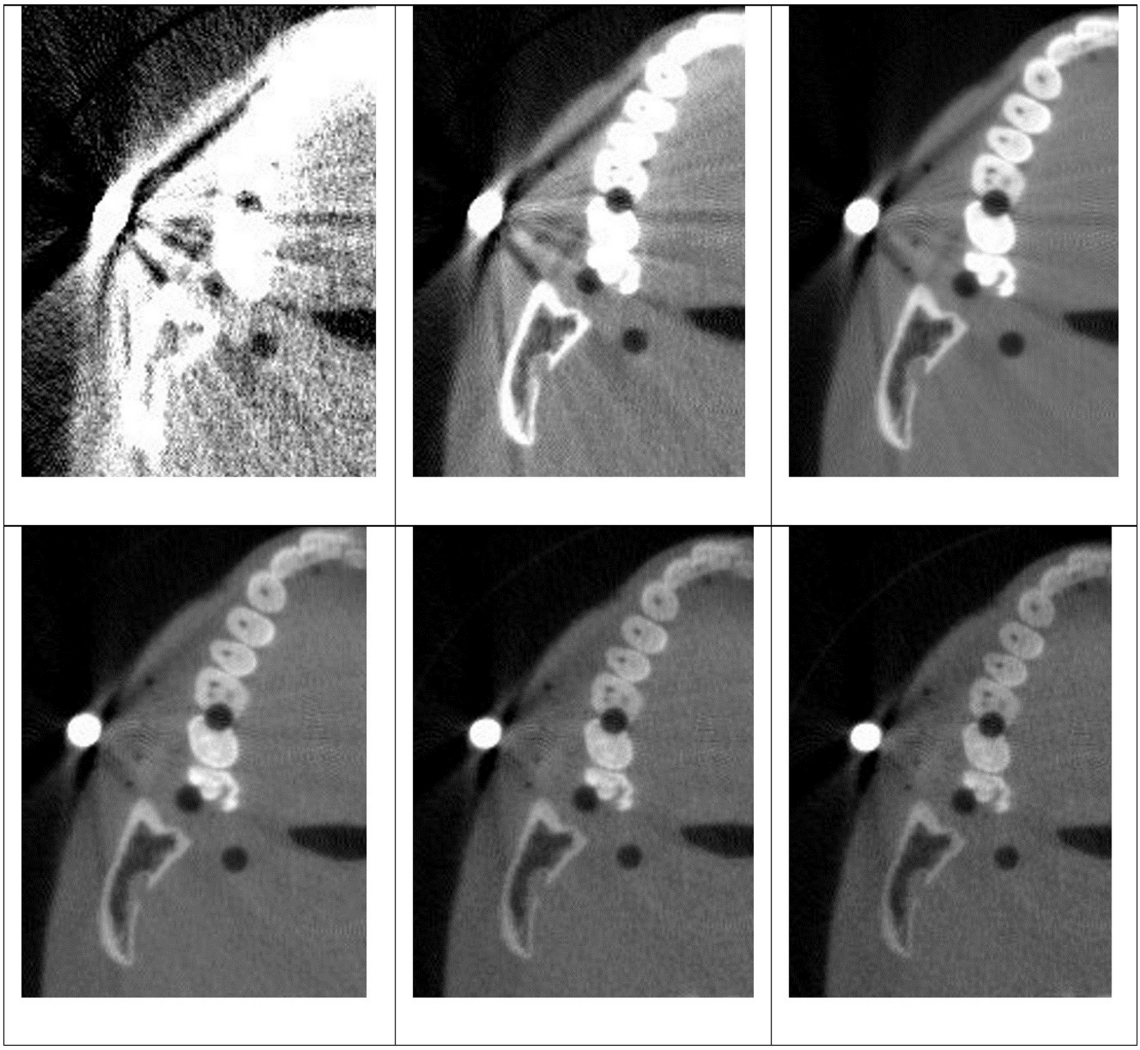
Region with the metal artifacts from the small metal bead. From left to right: Single-energy clinical CBCT, LE, HE, and VMI at 120KeV.
